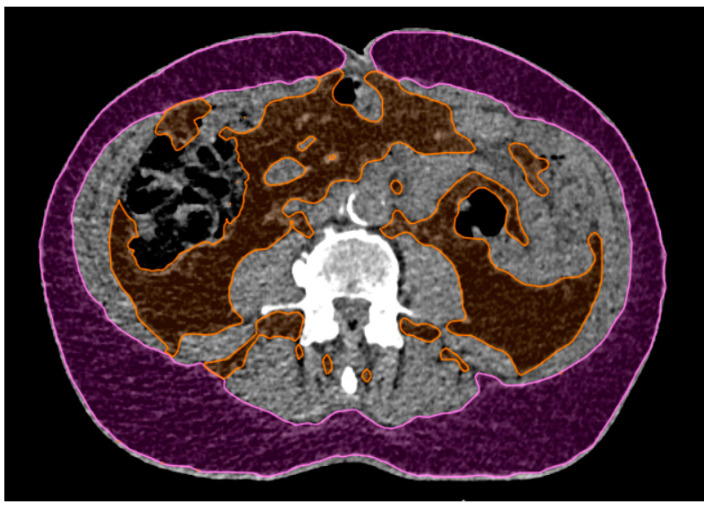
An example of subcutaneous (magenta) and visceral (orange) adipose tissue delineated on a single CT slice at the level of L4. Areas of the delineated surfaces relative to the total slice surface area were used to compute the subcutaneous, visceral and total adipose tissue percentages (SAT%, VAT% and TAT%, respectively).
